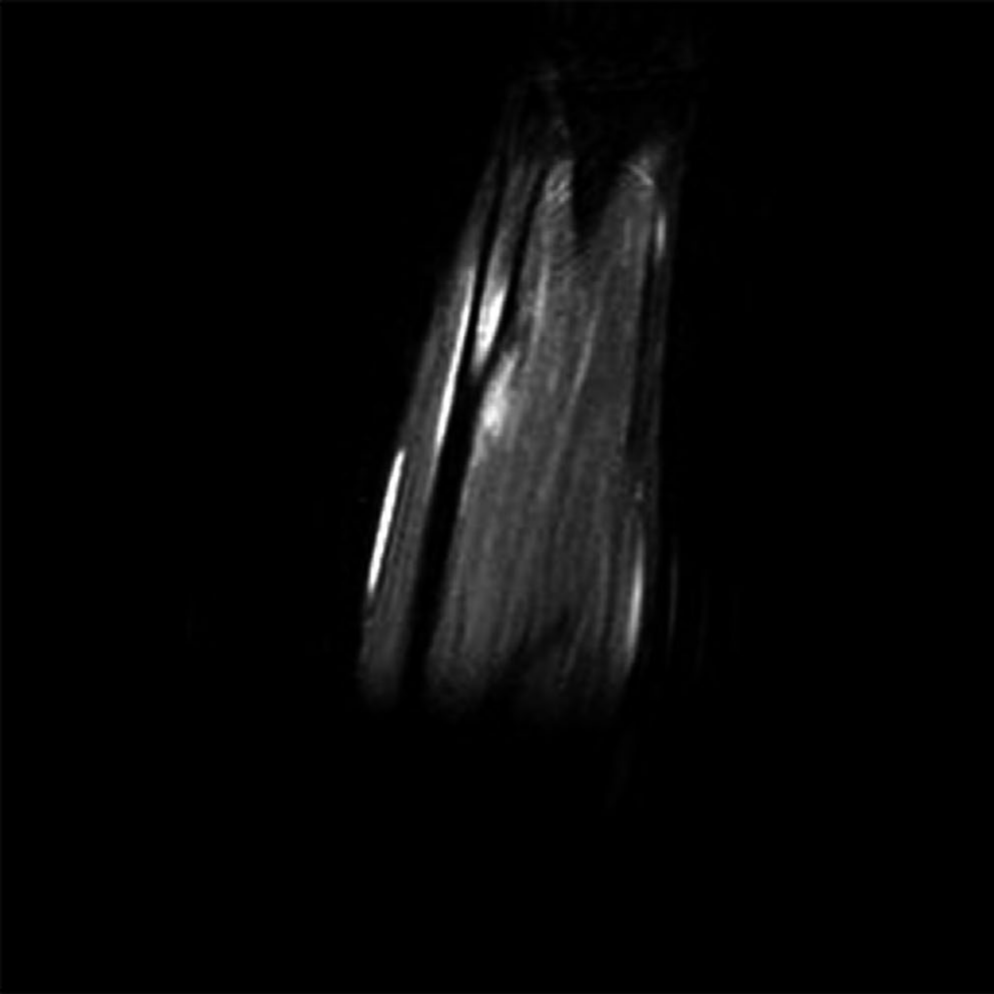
MRI without contrast, T2 image, coronal view
